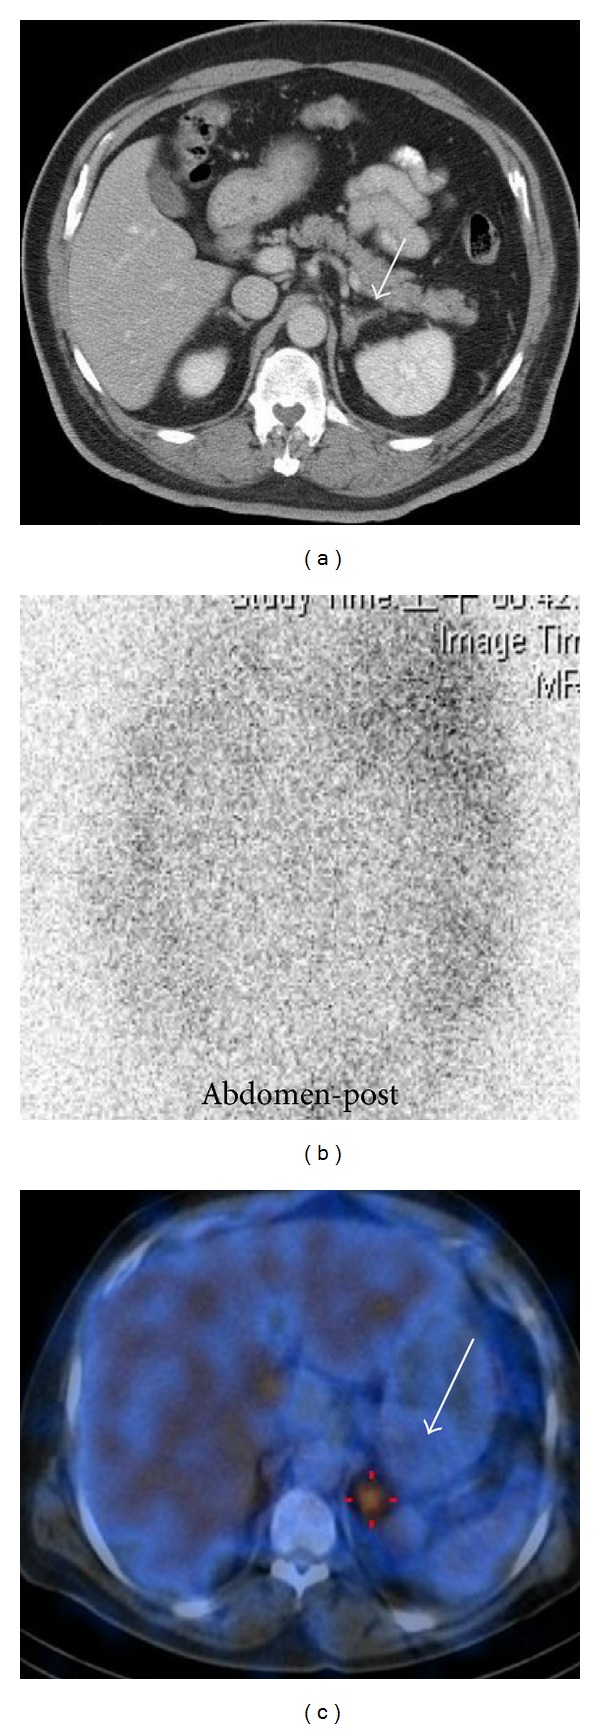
A 56-year-old male patient (case 4) with stage 1 hypertension for 3 years presents with atypical triad of PA and normokalemia. Contrast adrenal CT (b) shows a 1.2 cm nodular lesion in left adrenal gland (arrow). NP-59 96 h planar posterior imaging (b) indicates no radiotracer uptake within either adrenal gland, but fused SPECT/CT (c) indicate intense uptake in the left adrenal (arrow), consistent with left adrenal aldosteronism. His hypertension cured following left adrenalectomy.
